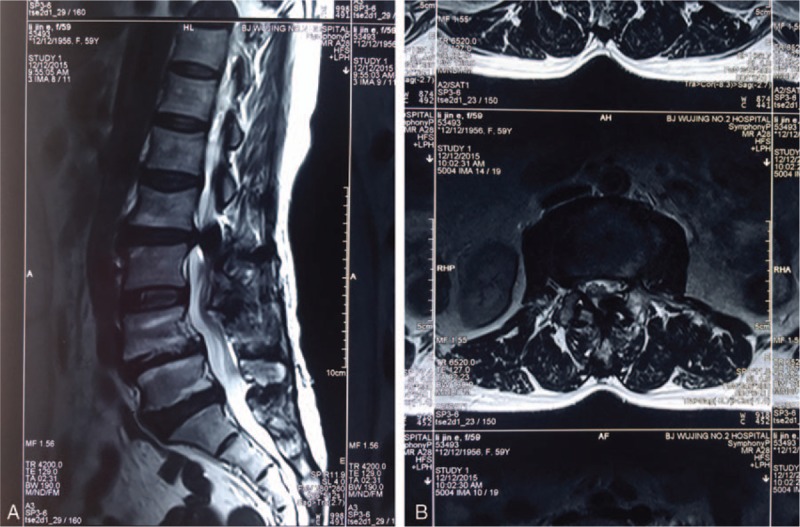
Magnetic resonance imaging shows (A) sagittal reconstruction and (B) an axial section shows intraspinal migration of the left rod.
